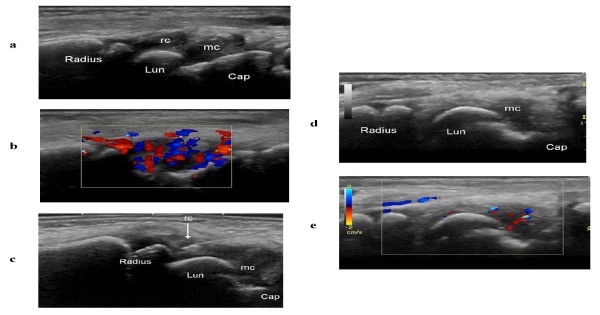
Dorsal sagittal US scans of the radio-carpal and midcarpal recesses. a, b Hypoechoic rounded radio-carpal (rc) and midcarpal (mc) recesses (a) with hyperemia on the color Doppler examination (b). Lun = lunate bone, Cap = capitate bone. c US-guided puncture with the tip of the needle (arrow) in the radio-carpal recess (rc). Lun = lunate bone, Cap = capitate bone, mc = midcarpal recess. d, e Four-week US follow-up of a midcarpal recess (mc) not treated with steroid injection, showing persistent synovial hypertrophy (d) and hyperemia (e). Lun = lunate bone, Cap = capitate bone.
